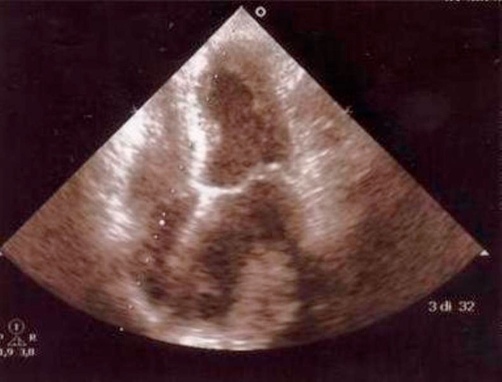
Transthoracic echocardiography (TTE) apical four chamber view shows an apparent left atrial “mass” in a dilated left atrium.
